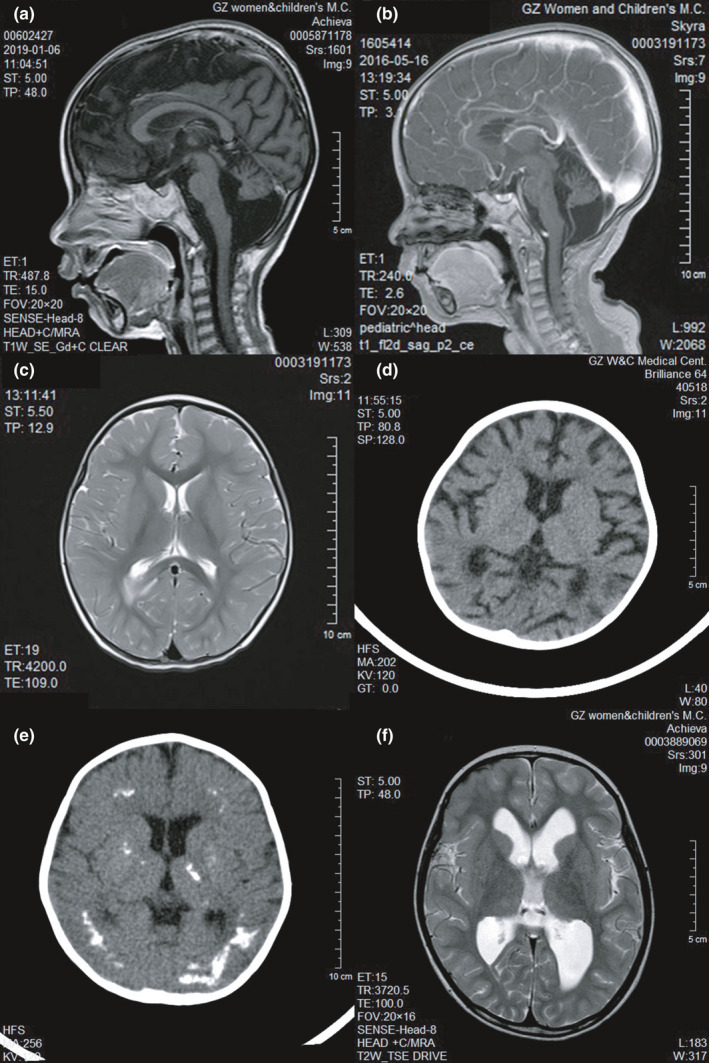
The patients' imaging features. (a) Patient 4, a median sagittal enhanced T1‐weighted image showing significant hypoplasia of the cerebellum with slightly enlarged fissure and enlargement of the posterior fossa. (b) Patient 3, cerebellar hypoplasia. (c) An axial T2‐weighted image of the bodies of the lateral ventricles in Patient 3 showing abnormal hyperintense signals in the white matter near the posterior horn of the right lateral ventricle and in the splenium of the corpus callosum. (d) An axial CT image of the horns of the lateral ventricles in Patient 4 showing deepening of the cerebral sulci in the two cerebral hemispheres and mild widening of the two lateral ventricles, suggesting brain atrophy. (e) A CT image of Patient 1 showing symmetrical, irregularly shaped calcifications in the two frontal lobes, the occipital lobe, and the basal ganglia. (f) An axial T2‐weighted image of Patient 2 showing dilation of the two lateral ventricles and the third ventricle, suggesting hydrocephalus
